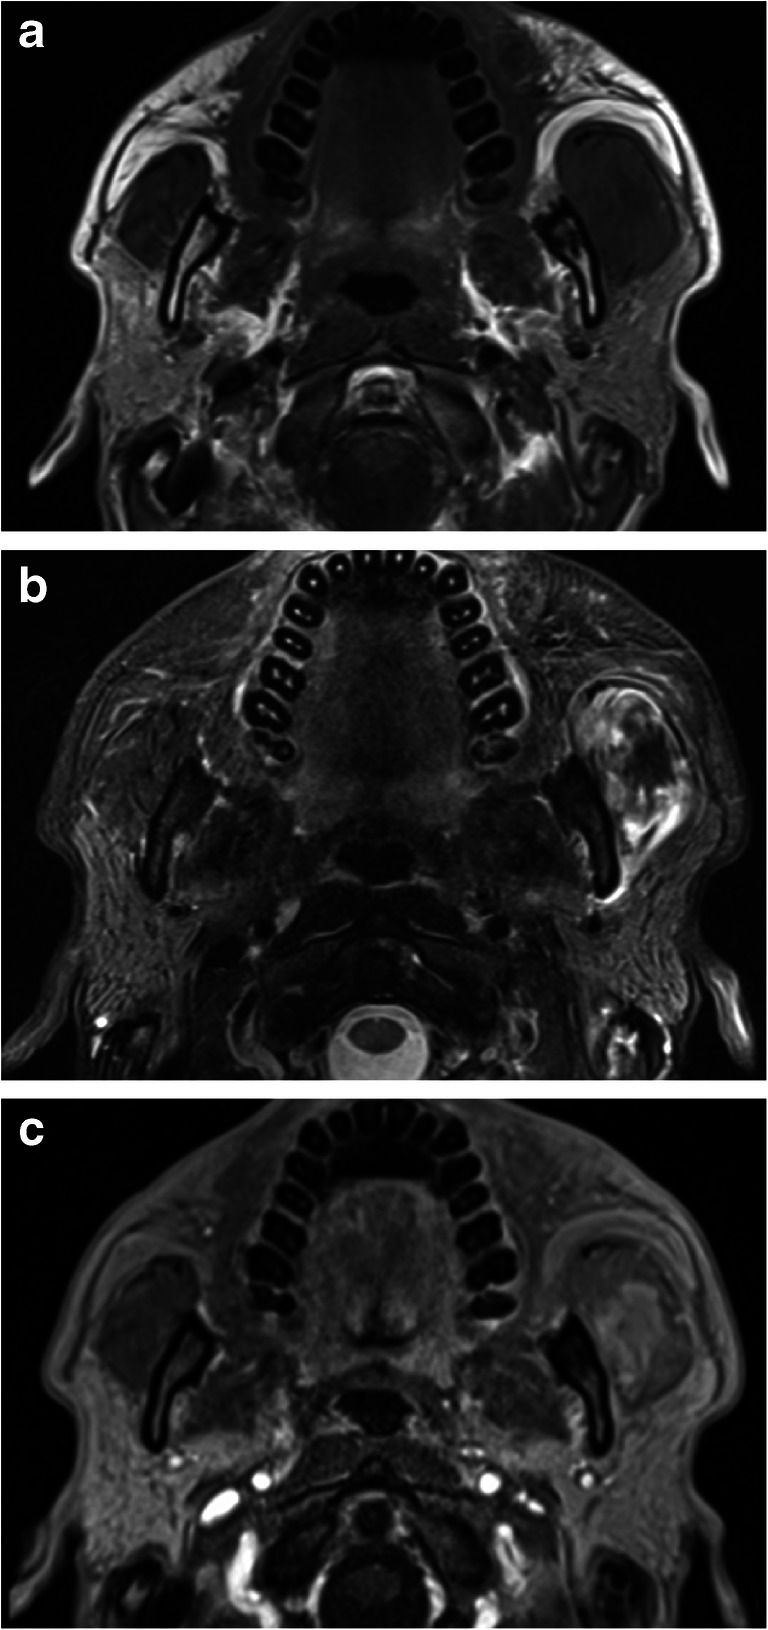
Axial magnetic resonance images. a The T1-weighted image shows swelling of the left masseter with isointensity to the muscle. b The fat-suppressed T2-weighted image shows swelling of the left masseter muscle with an apparent increase in signal intensity in the periphery and a focal mass-like or reticular low signal intensity in the center. c Dynamic contrast-enhanced image 30 s after injection of the contrast agent shows high enhancement in the central mass-like area of the left masseter muscle
